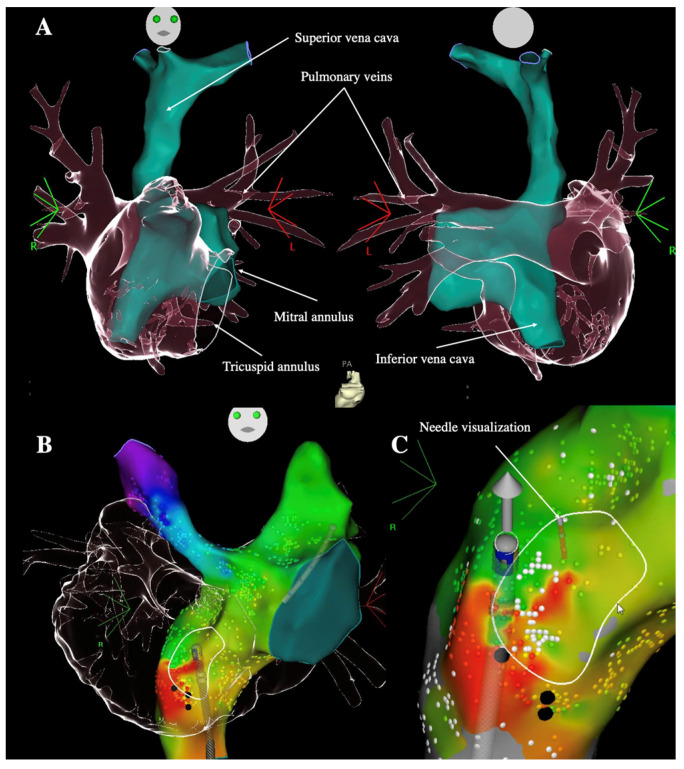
Transbaffle puncture in patients with D-TGA and atrial switch. (A) CT-scan images of a patient with Senning surgery in right anterior oblique 30° (left) and posteroanterior view (right) showing that the systemic venous atrium is surrounded by the pulmonary venous atrium. In another patient with Mustard surgery, after the merging of electroanatomical and CT-scan images, the optimal site for transbaffle puncture is located (white circle, (B)) and the transseptal needle is connected to be visualized (C) (anteroposterior views).
